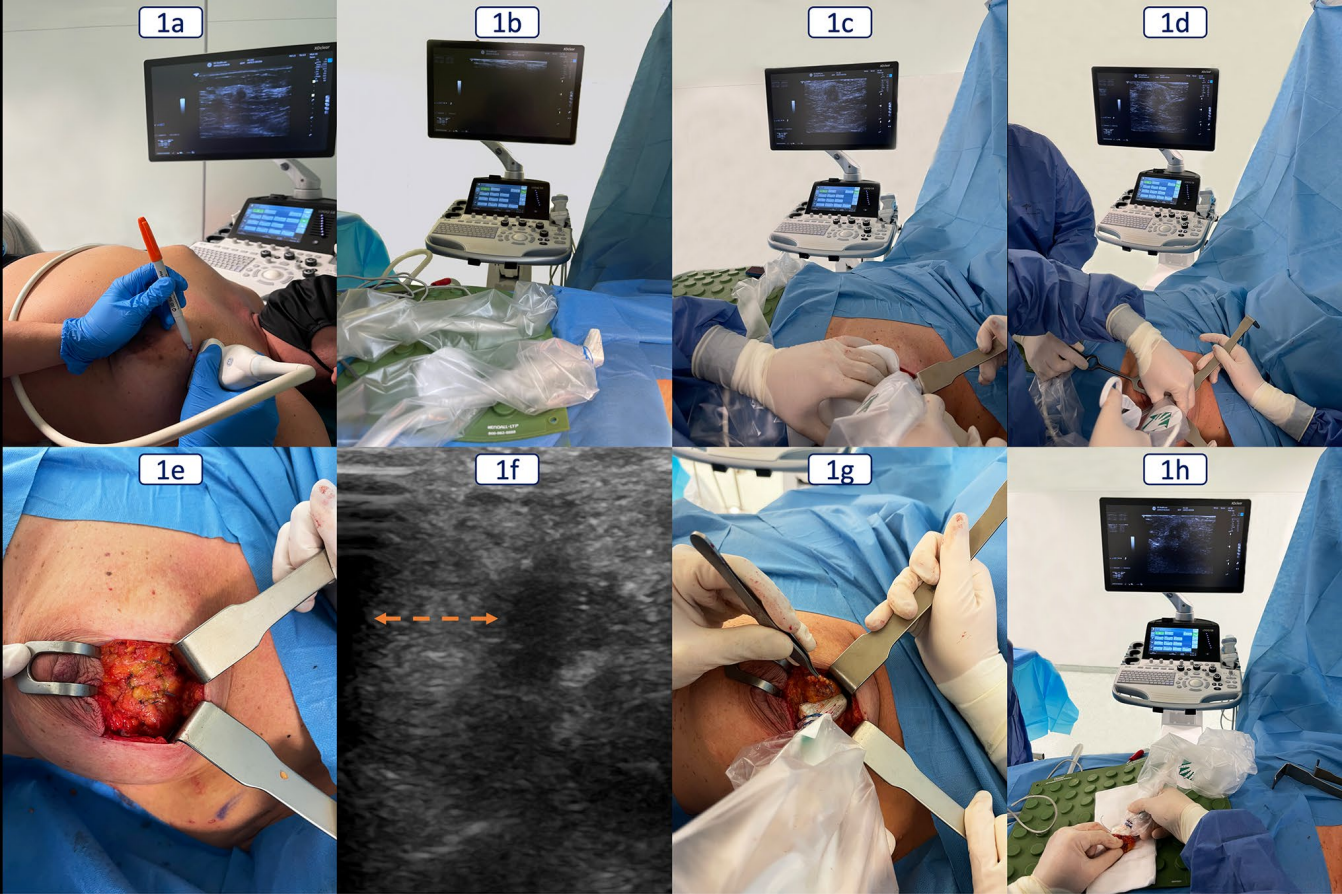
Intra-Operative Ultrasound-guided Surgery (IOUS) personal technique
